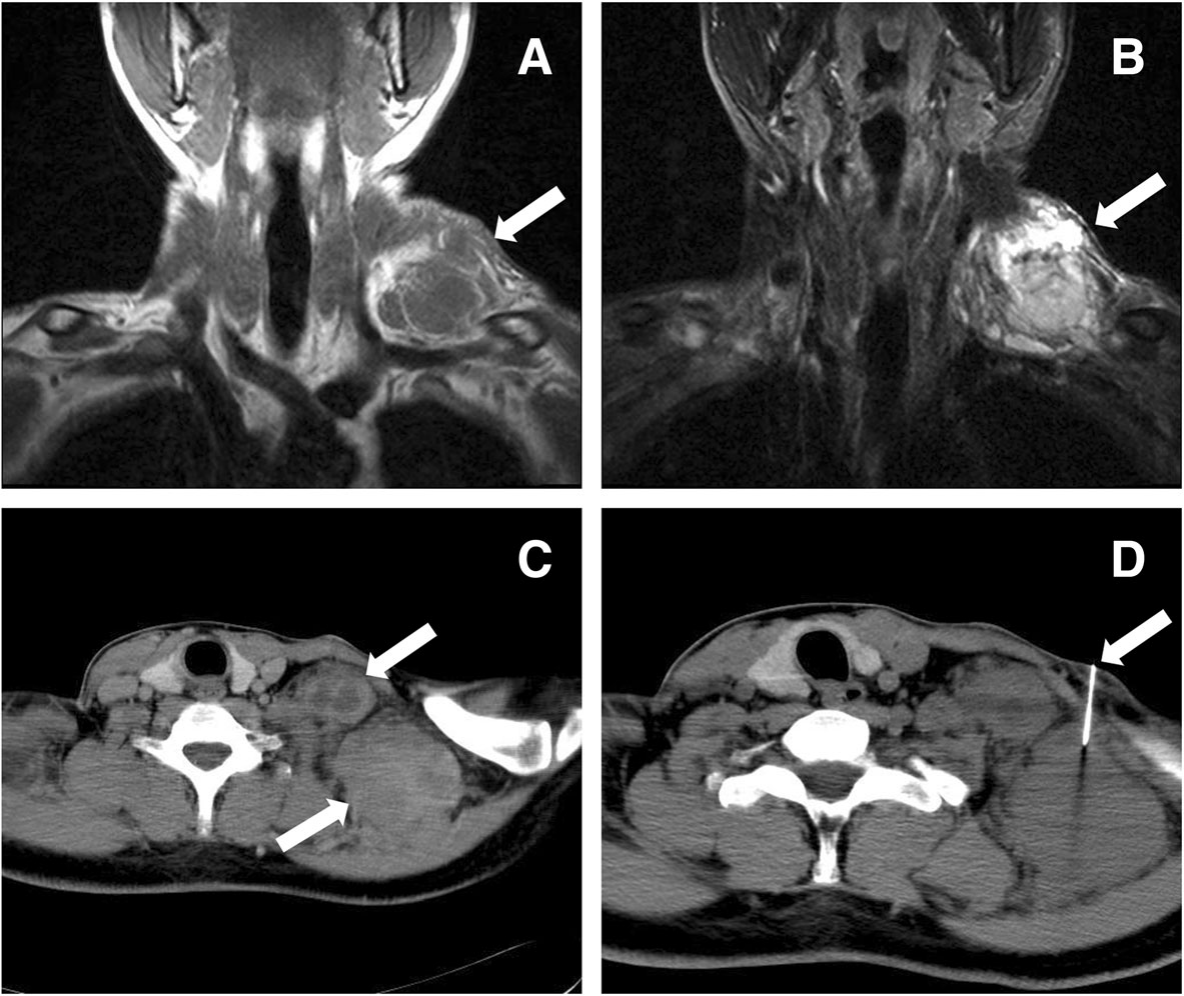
T1-weighted images (a) and T2-weighted coronal images (b) on MRI show a soft tissue mass with inhomogeneous densities and fine separations in the left supraclavicular fossa (arrows). Axial CT scan (c) reveals a fused multinodular mass (arrows) with local necrosis; CT-guided core needle biopsy was then performed (d)
